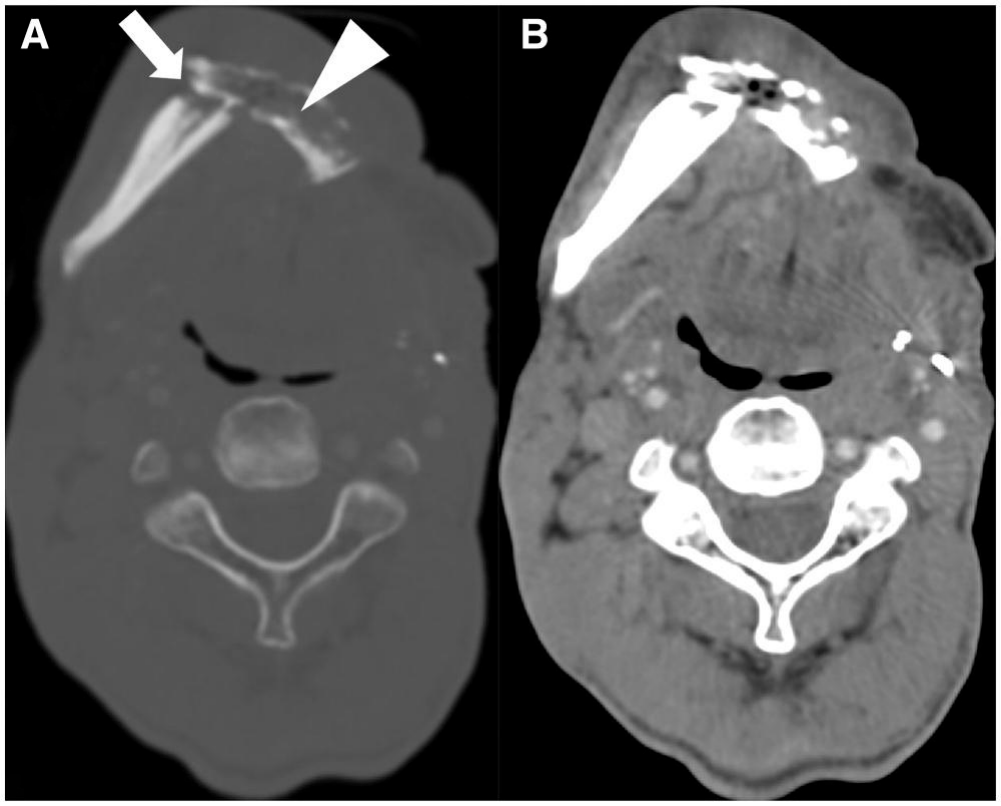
CT with bone algorithm (A) and CECT in soft tissue window (B) in a post-left hemimandibulectomy and post adjuvant chemoradiotherapy (CRT) case of lower gingivobuccal carcinoma shows fractured fragment (arrow) involving body of mandible with multiple lucencies (arrowhead) and without any suspicious enhancing soft tissue, suggestive of osteoradionecrosis (delayed complication of RT).
